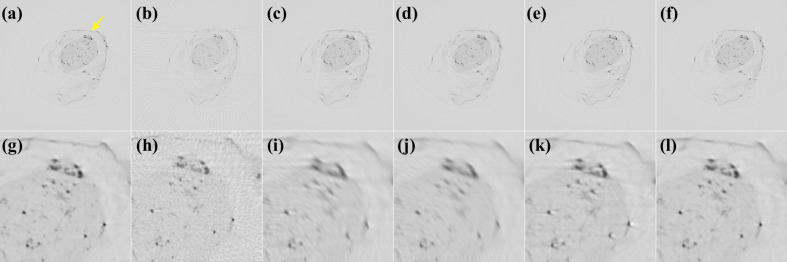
Tomographic images of *Drosophila*. (*a*) The ground-truth image and images reconstructed from 75 X-ray projection views (*b*) directly or with synthesis by (*c*) bicubic interpolation, (*d*) *CyclicGen*, (*e*) bicubic interpolation with RU-Net, or (*f*) *CyclicGen* with RU-Net. (*g*)–(*l*) Magnifications of the region indicated by a yellow arrow in (*a*) for the corresponding figures in the first row.
